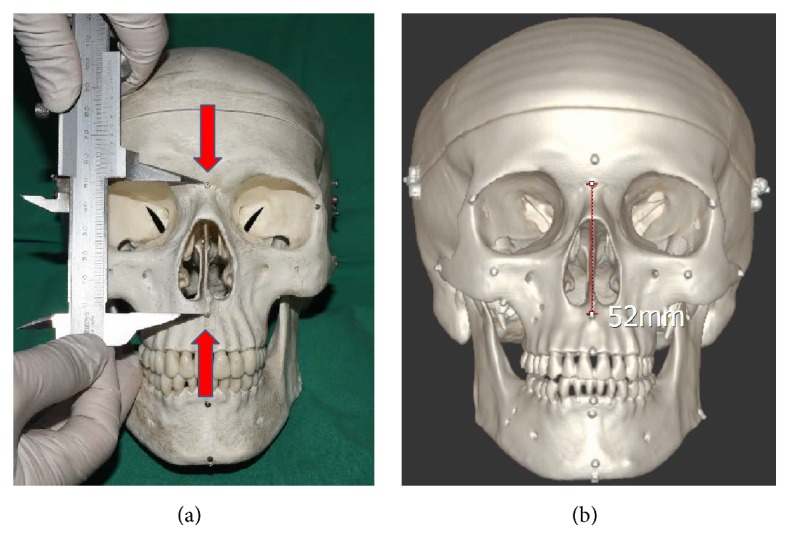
Physical and CT-based measurements of skull models. (a) The five set linear distances were measured using Vernier calipers (real values). (b) The values of the same distances were measured based on the PACS, which was obtained via 3D reconstruction of CT scans (PACS measurement).
